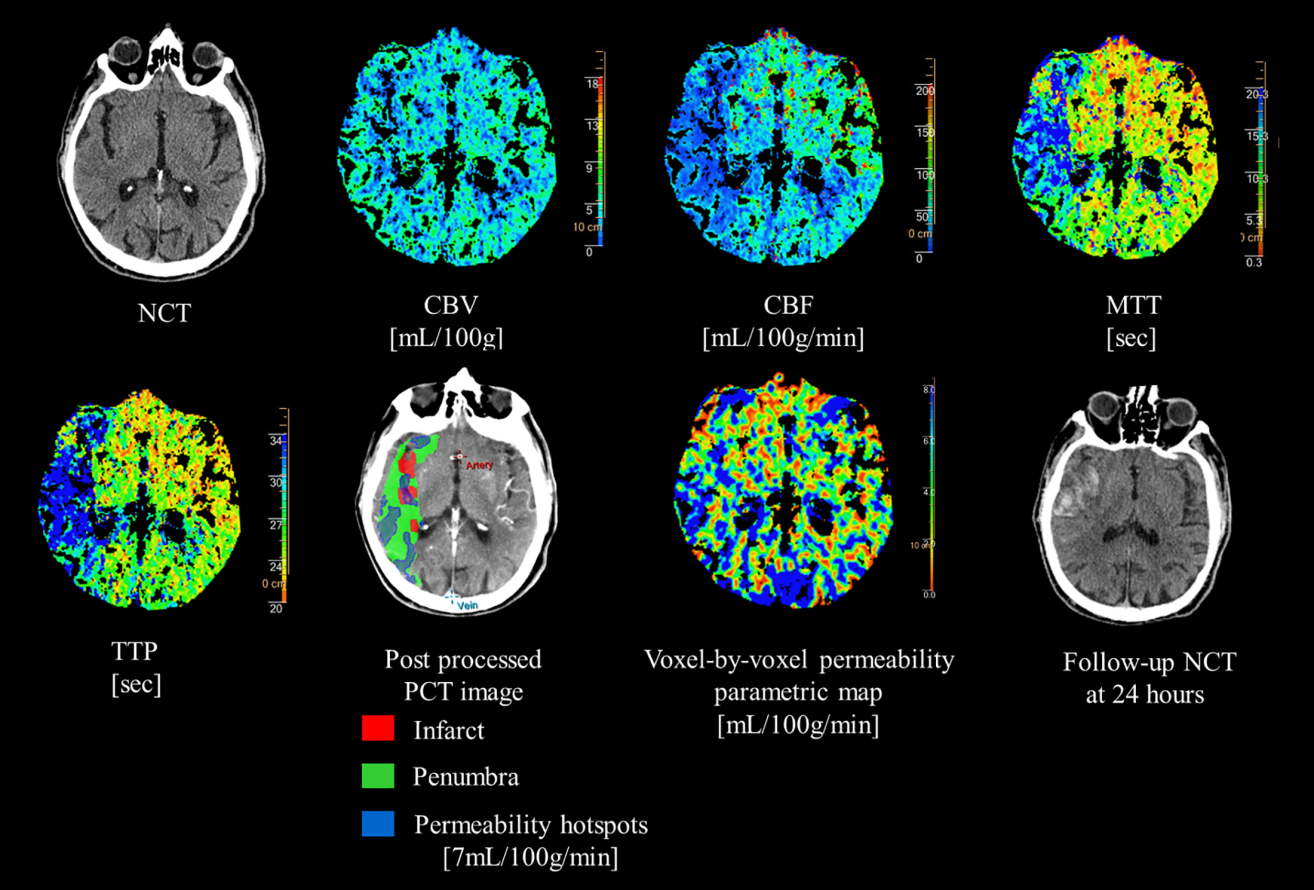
Perfusion CT protocol in a patient with PH-2 in the ischemic right MCA territory following intravenous thrombolysis. Post-processed map shows a large penumbra in right MCA territory. Note the clearly increased baseline permeability at thresholds of 7 ml/100g/min in the area of the parenchymal hematoma.
